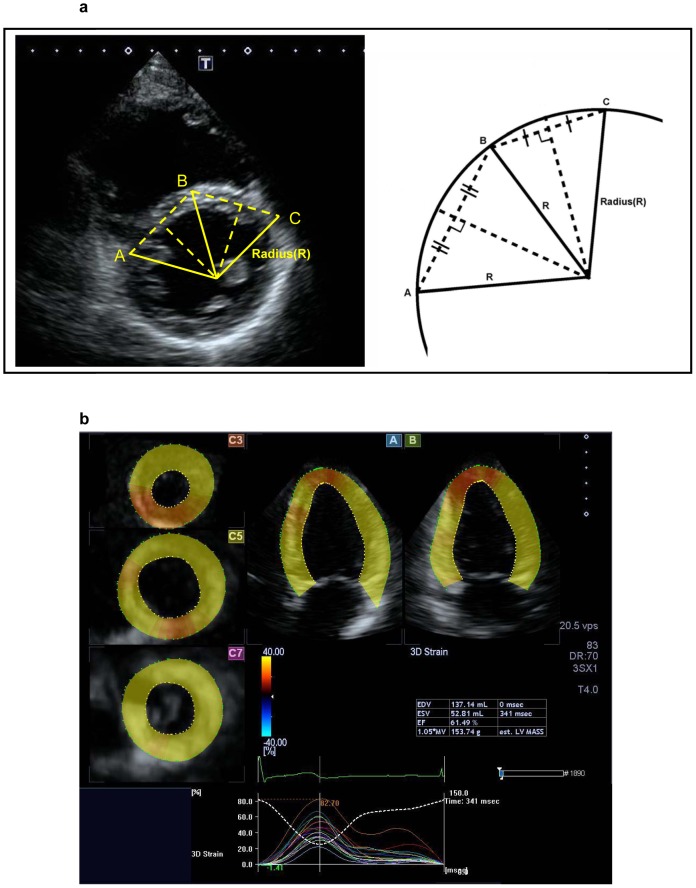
Two-dimensional echocardiographic assessment of ventricular septal configuration and 3D speckle tracking analysis. (a) The radius was identification from the intersection of perpendicular bisectors between the anterior (C) and middle (B) and between the posterior (A) and middle (B) points in the ventricular septum. (b) The 5-plane view used for 3D speckle tracking analysis: A plane (apical 4-chamber view), B plane (2-chamber view), and 3 C planes (short-axis views near the apex, at mid-level, and at the base of the left ventricle).
